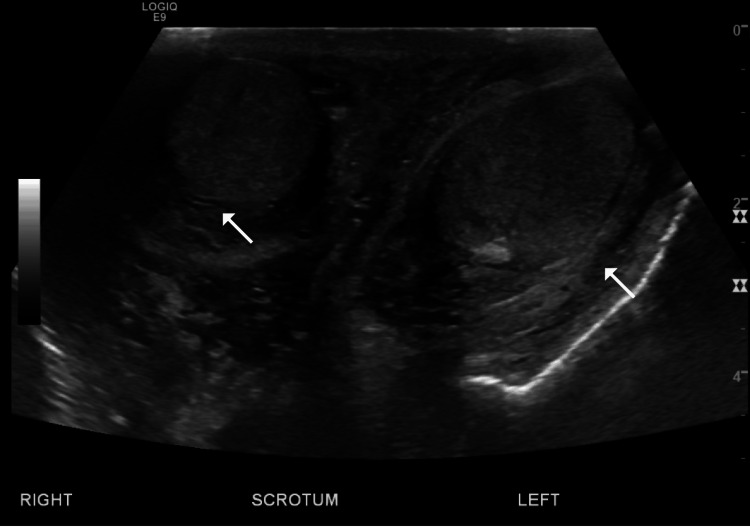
Bilateral scrotal edema Scrotal edema is characterized by an "onion skin" pattern on ultrasound, where the thickened scrotal wall is striated or layered in appearance, as indicated by arrows.
